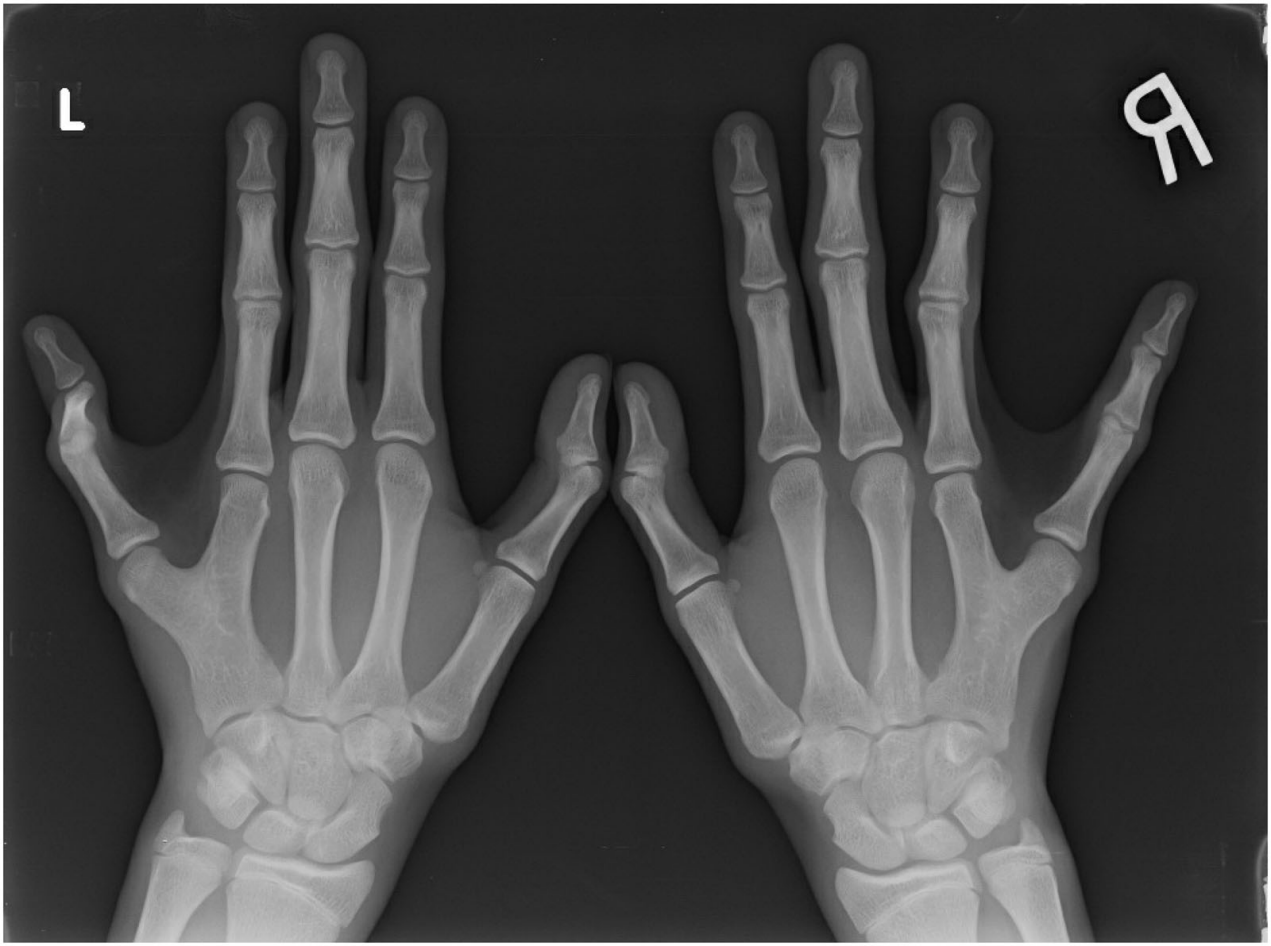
Preoperative radiographs of the patient’s left and right hands.
